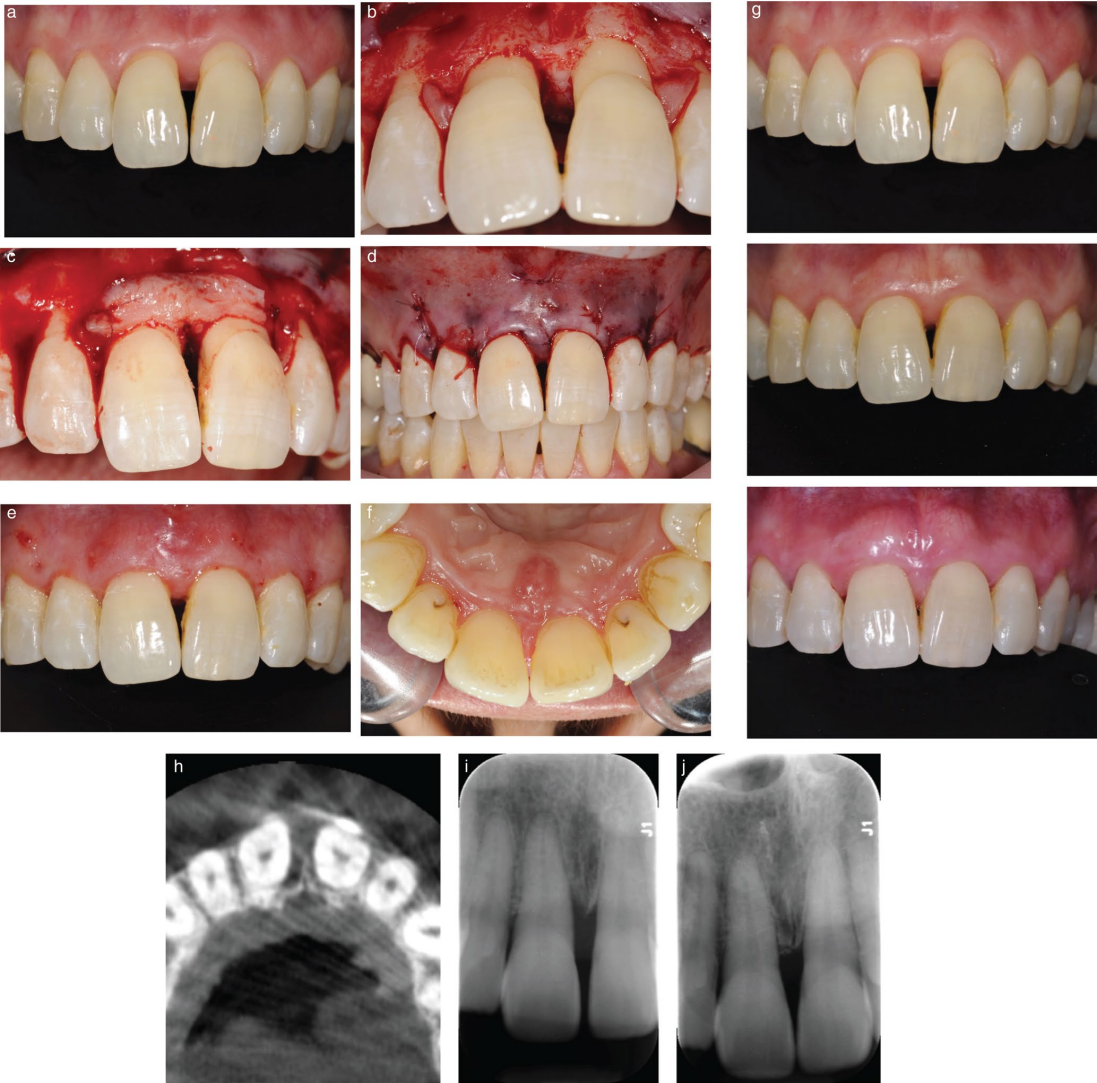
Case 1 (a) Preoperative view showing loss of the papilla, buccal recession, and a diastema. Infrabony defect following granulation tissue removal (b), placement of a contoured CTG (c), and flap closure (d). (e, f) Postoperative views at 15 days on the buccal and palatal aspects, showing maintained primary closure. (f) Soft tissue outcomes from baseline to 12 and 56 months, showing complete coverage of the buccal recession, an increase in papilla height, and spontaneous closure of the diastema. (g–i) Radiographic follow‐up (baseline CBCT and periapical radiographs at baseline and 56 months) showing complete bone fill.
